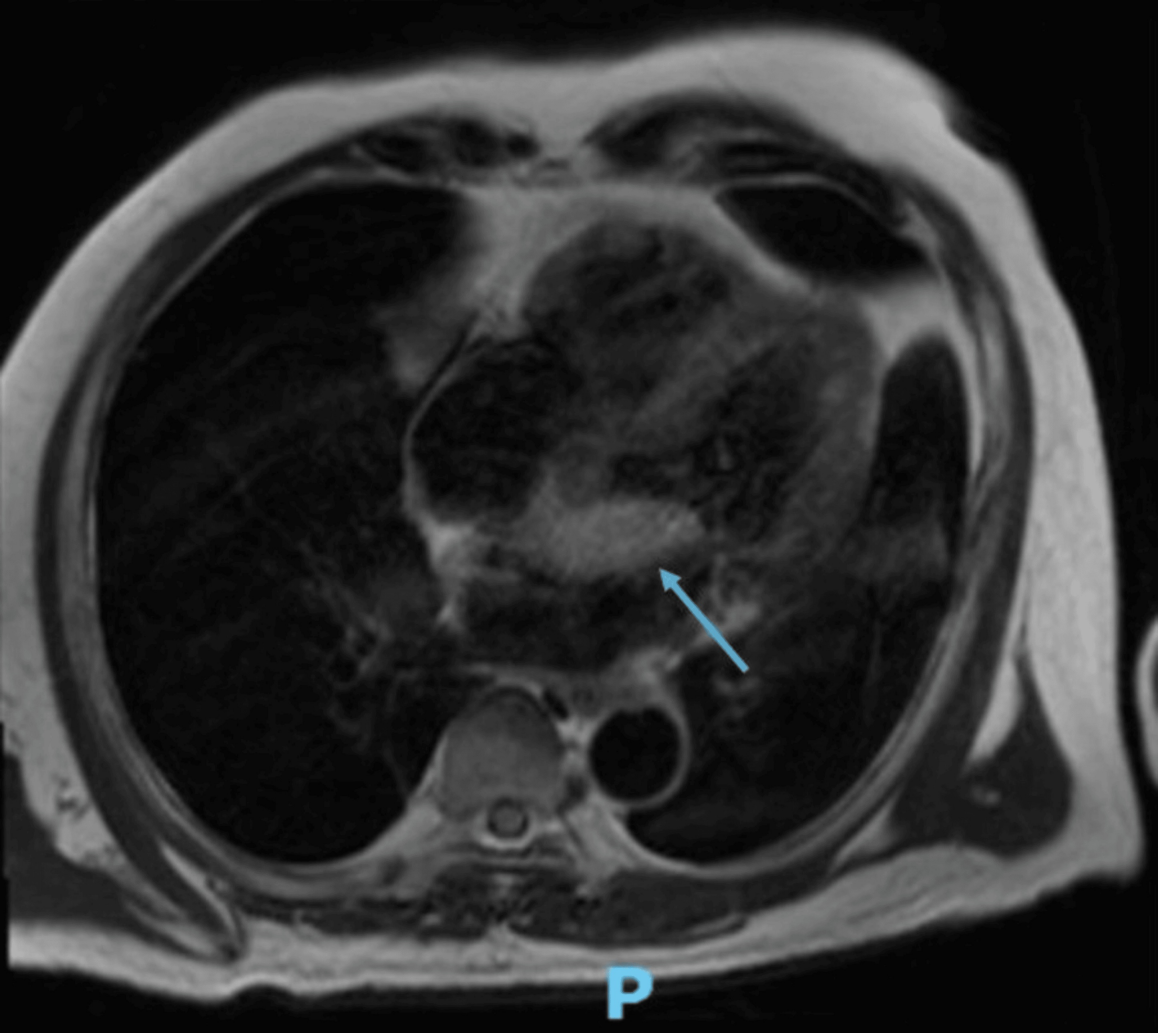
Cardiac MRI image in T2 sequence. The blue arrow indicates the location of a narrow-based left atrial mass measuring 4.3 x 1.9 cm arising from the interatrial septum.
